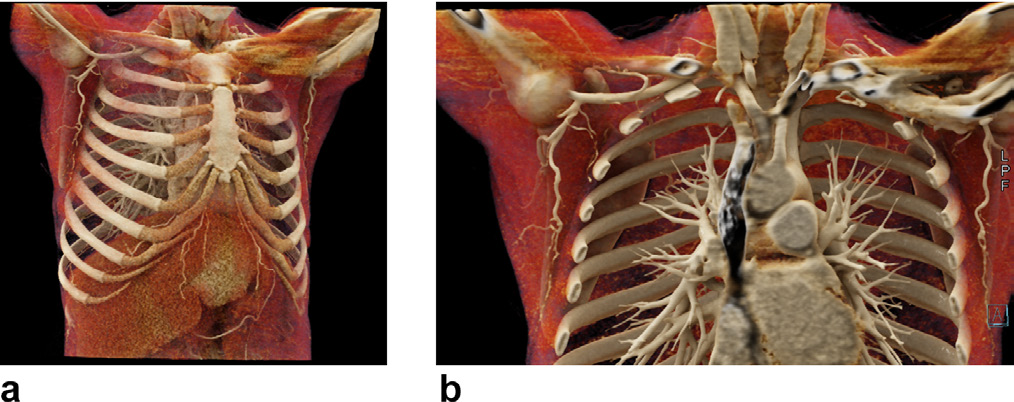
49-year-old transgender patient presented to the hospital for a pre-surgical evaluation of a TDAP phalloplasty. CTA imaging of the chest and evaluation with CVRT revealed well-defined, bilateral thoracodorsal arteries (big arrows) and its perforators (small arrows). (A) Right anterior oblique projection view. (B) Anterior projection view. CTA, CT angiography; CVRT, cinematic volume rendering technique; TDAP, thoraco dorsal artery perforator.
